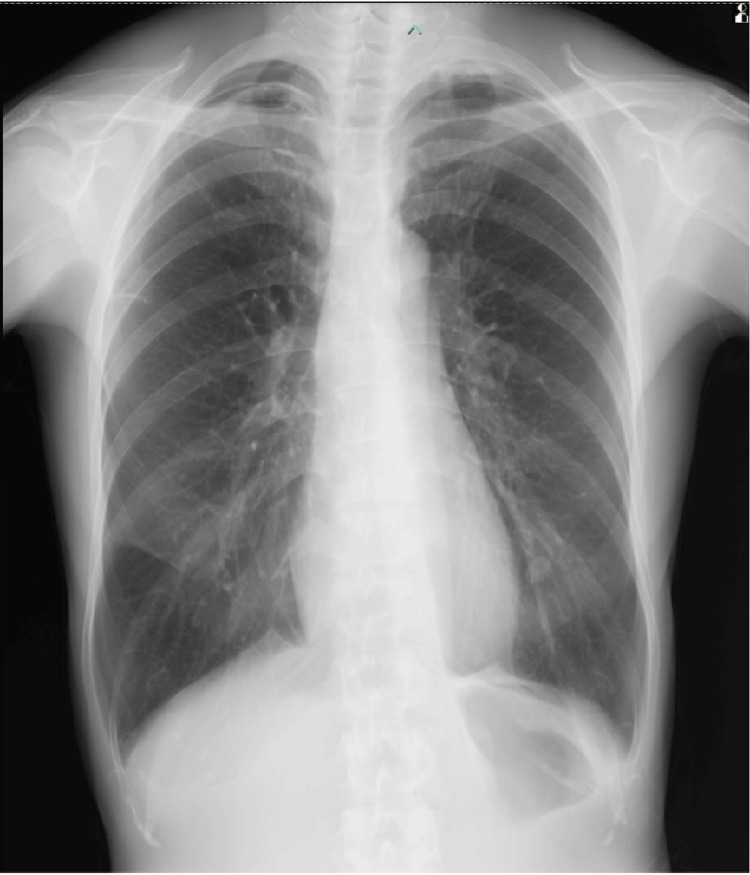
Chest radiography on postoperative day 30 Although space remained in the right lung apex, expansion of the right lung improved
